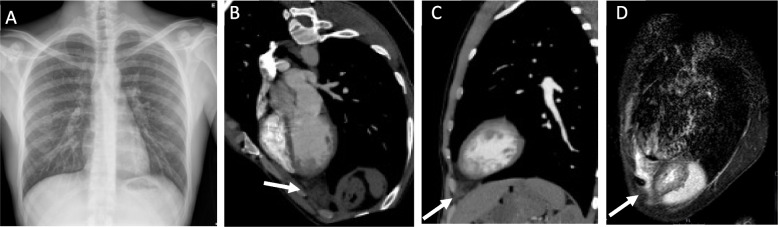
A – Normal chest radiograph; B and C – Contrast-enhanced chest CT reformatted axial and sagittal oblique images of the mediastinum demonstrate an ovoid-shaped mediastinal fat lesion with soft tissue stranding, consistent with necrosis of the epicardial fat at the left cardiophrenic angle (white arrows). D – Chest MRI sagittal oblique fluid sensitive T2 sequence image shows a fatty lesion with increased signal (edema) stranding in the left cardiophrenic angle (white arrow)
